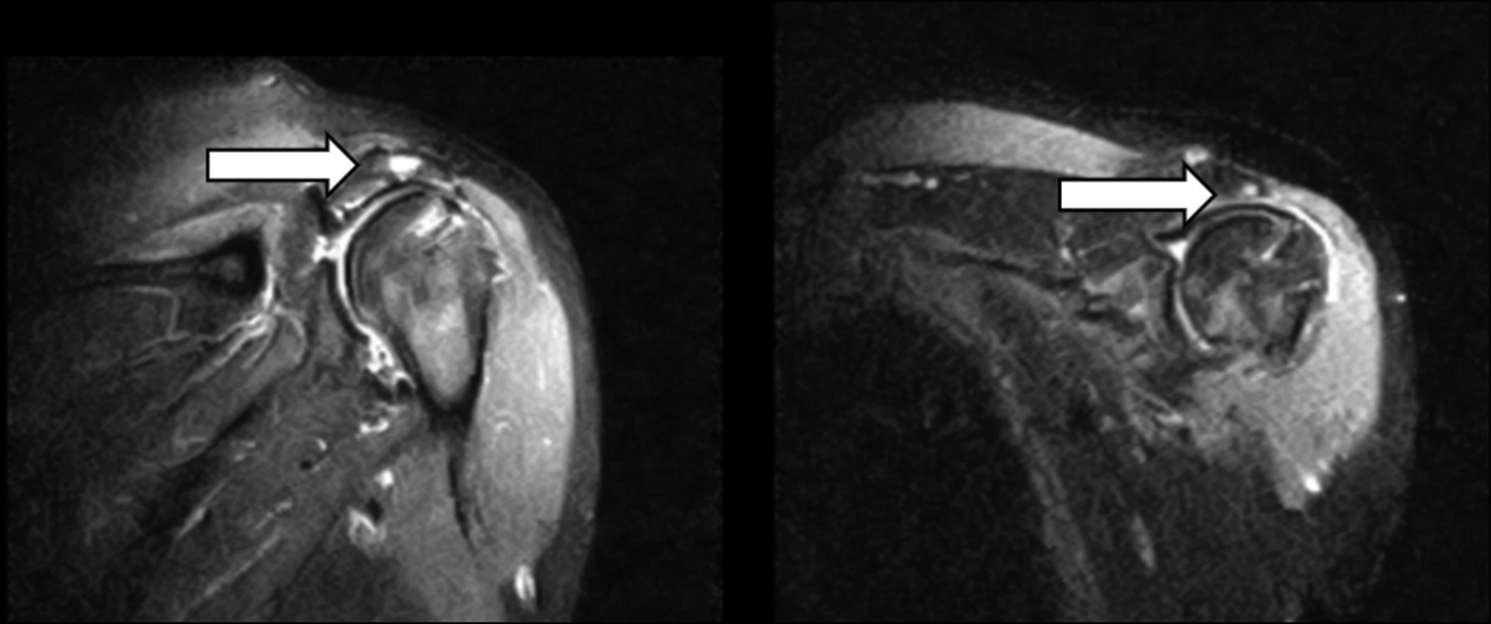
Images depicting bony edema. These images display postoperative MRI bony changes noted after superior capsular reconstruction. The arrows note bony edema at the level of the acromion after SCR. No SAD/acromioplasty was performed at time of procedure. This is likely secondary to continued abnormal contact between the humeral head and acromion. Both figures are T2 inversion recovery coronal images. (MRI, magnetic resonance imaging; SAD, subacromial decompression; SCR, superior capsular reconstruction.)
